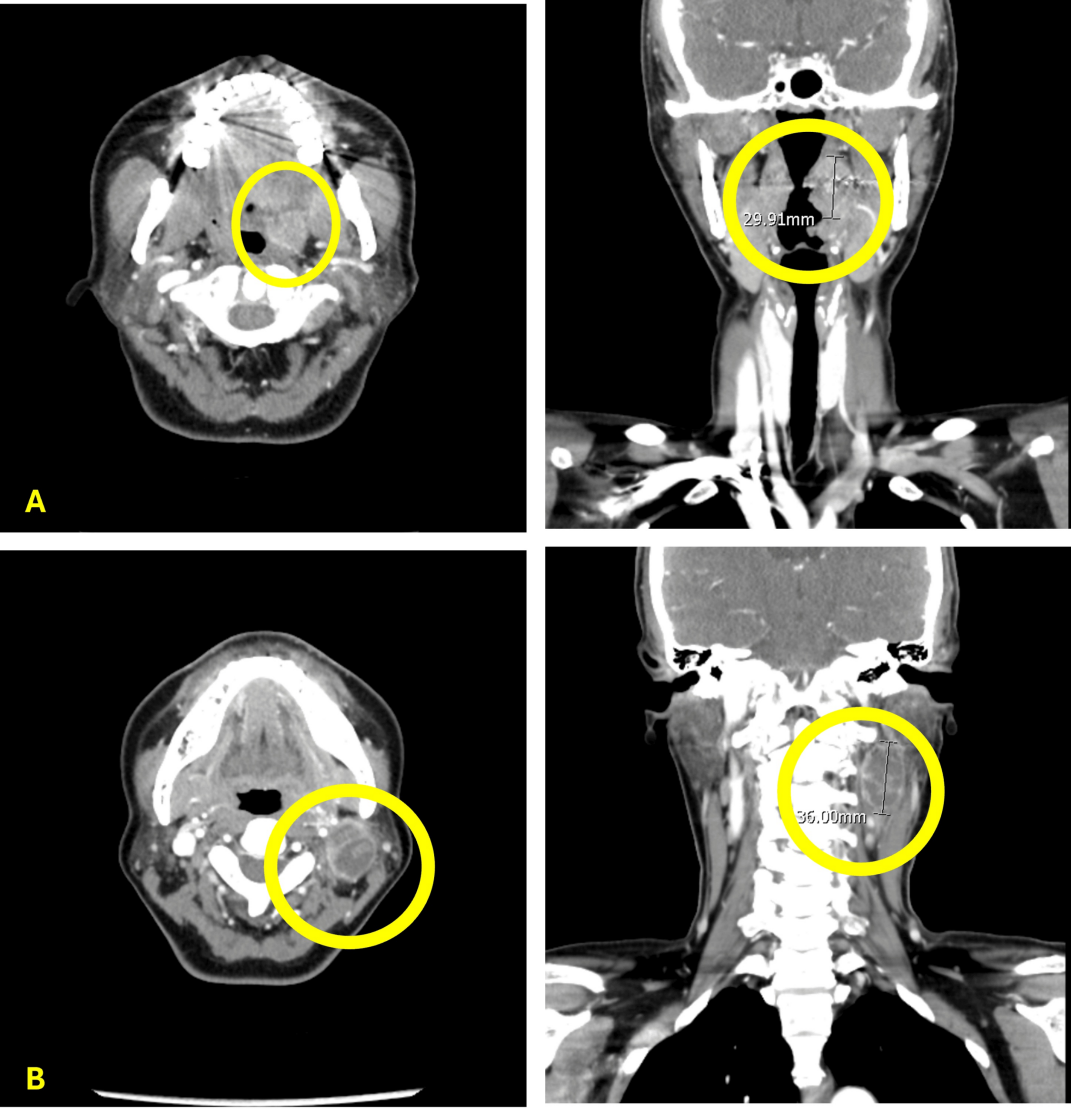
CT findings before the induction chemotherapy A.The images on the upper panel revealed an asymmetrically enlarged left tonsil, approximately 3 cm in its largest diameter, suspicious for tonsillar carcinoma. B. The images on the lower panel revealed a 3.6 cm cystic degenerative change at the right level II neck region, suspicious for lymph node metastasis CT: computed tomography
